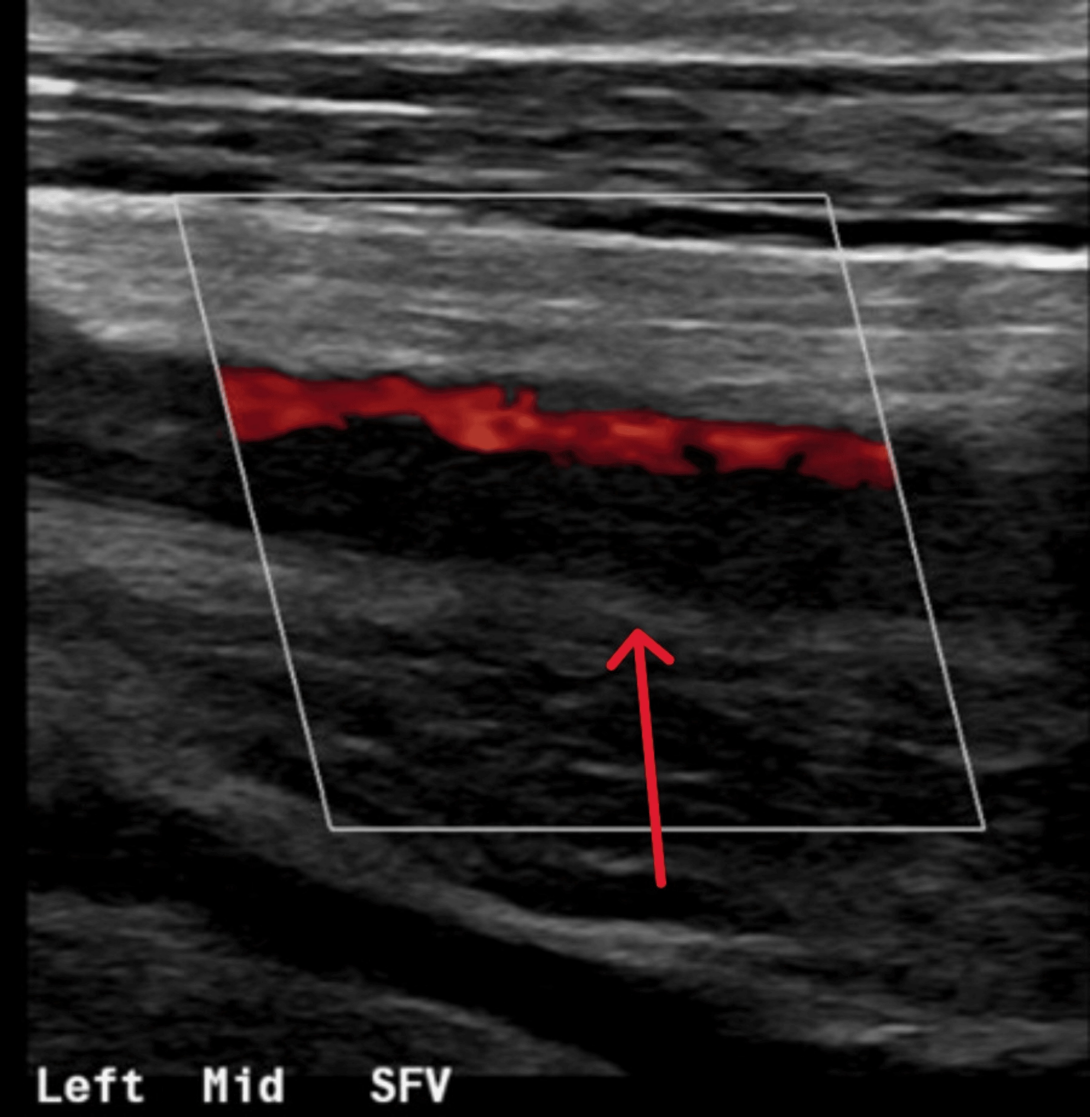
Doppler ultrasound of the left thigh showing deep vein thrombosis of the superficial femoral vein (red arrow)
